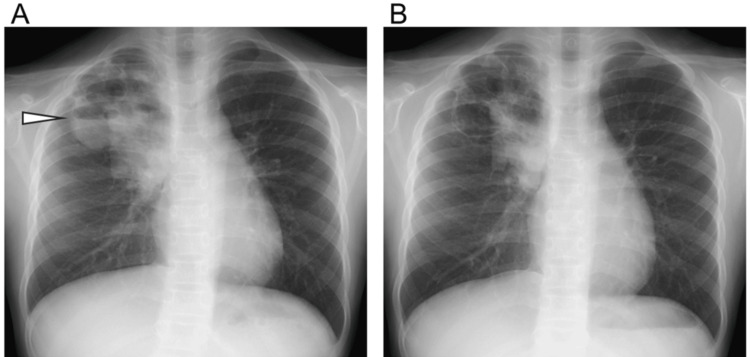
Chest X-ray images (A) On hospital day 11, infiltrative shadows and cavitary lesions were observed, with a fluid level visible within the cavitary lesion (arrowhead). (B) On hospital day 19, the infiltrative shadows had decreased in size but remained present, and the fluid level within the cavitary lesion had disappeared.
